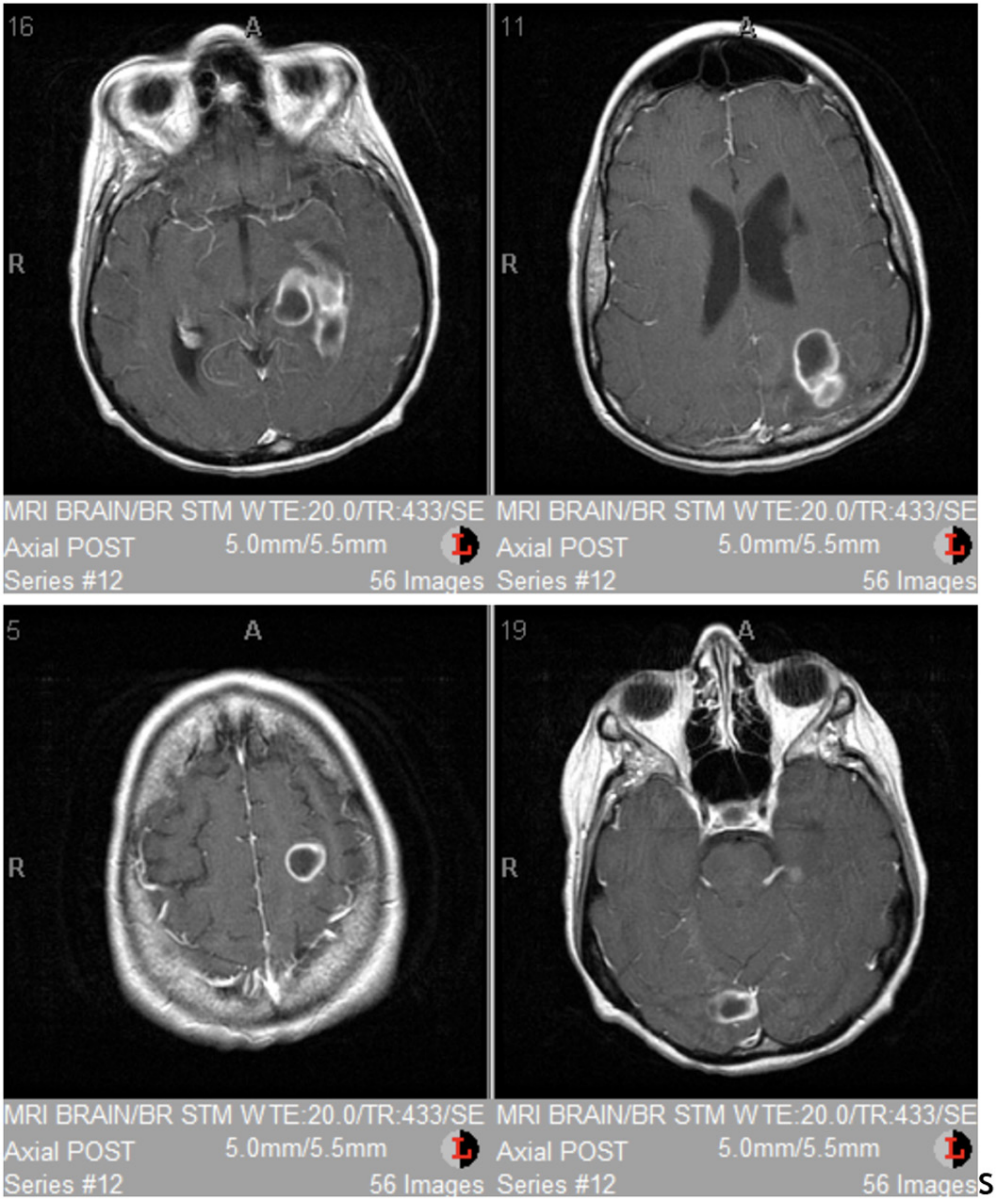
Brain MRI demonstrated intra‐axial ring‐enhancing lesions in the left posterior frontal lobe, left posterior parietal lobe, right occipital lobe and left temporal lobe, all with restricted diffusion and surrounding edema.
